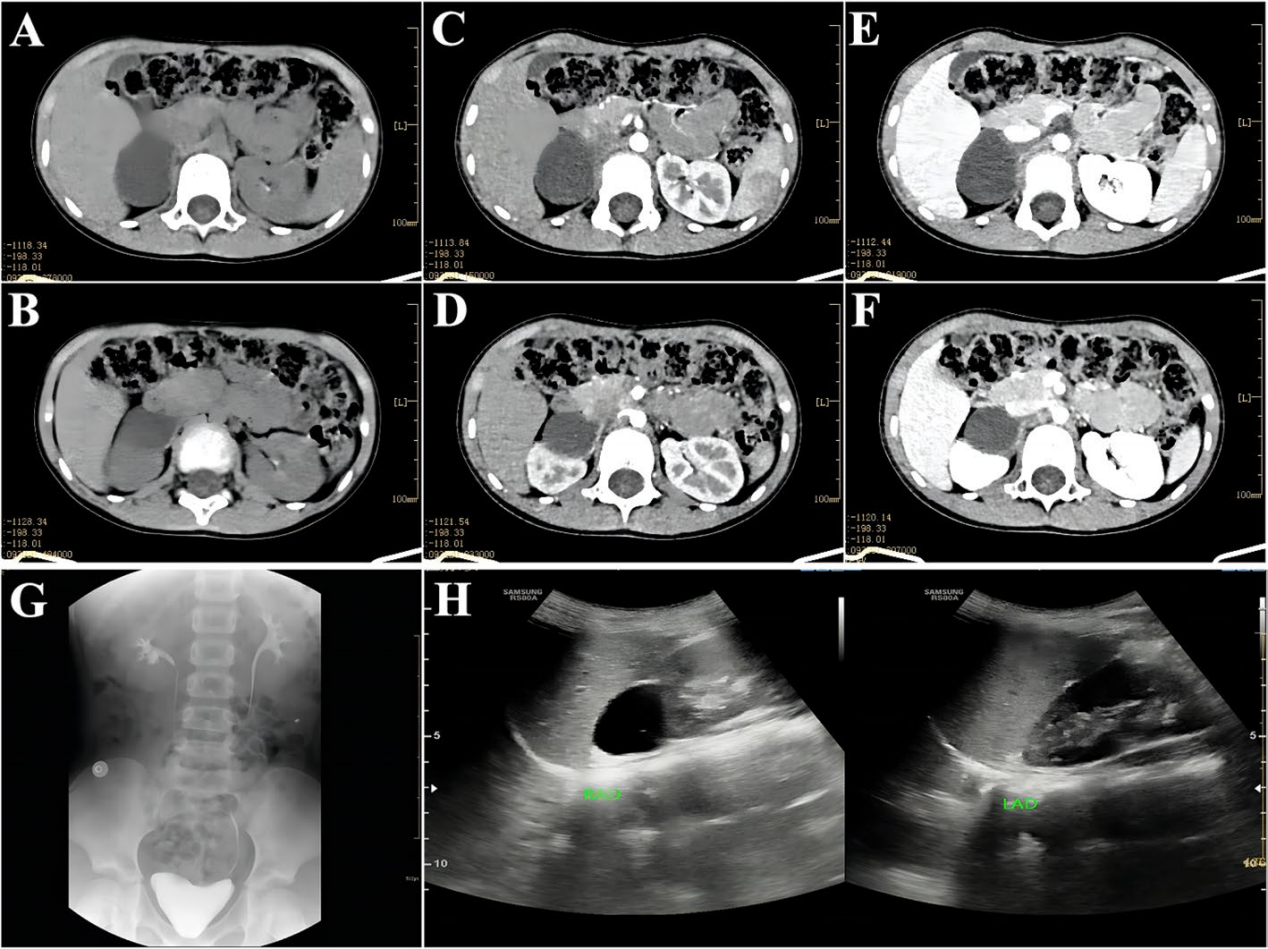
Preoperative imaging findings; A-B: adrenal CT scan findings; C-D: findings in the arterial phase of adrenal enhanced CT; E-F: findings in the venous phase of adrenal enhanced CT; G: findings of intravenous urography (IVP); H: Findings on color Doppler ultrasound of the adrenal gland
